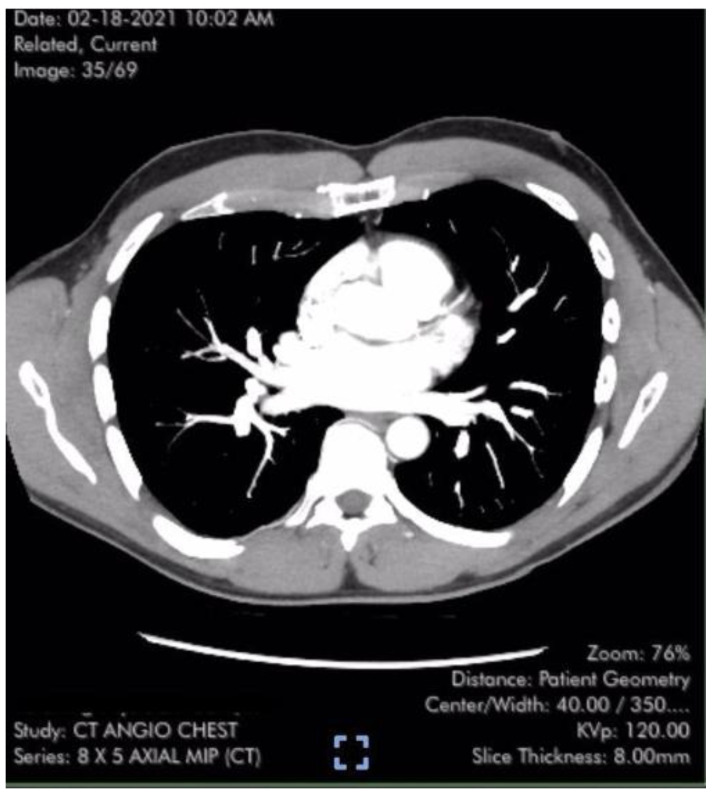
Axial CT image of the Case #1 patient from normal 3-dimensional pulmonary artery reconstruction without evidence of arterial clots or pulmonary emboli [6,7].
